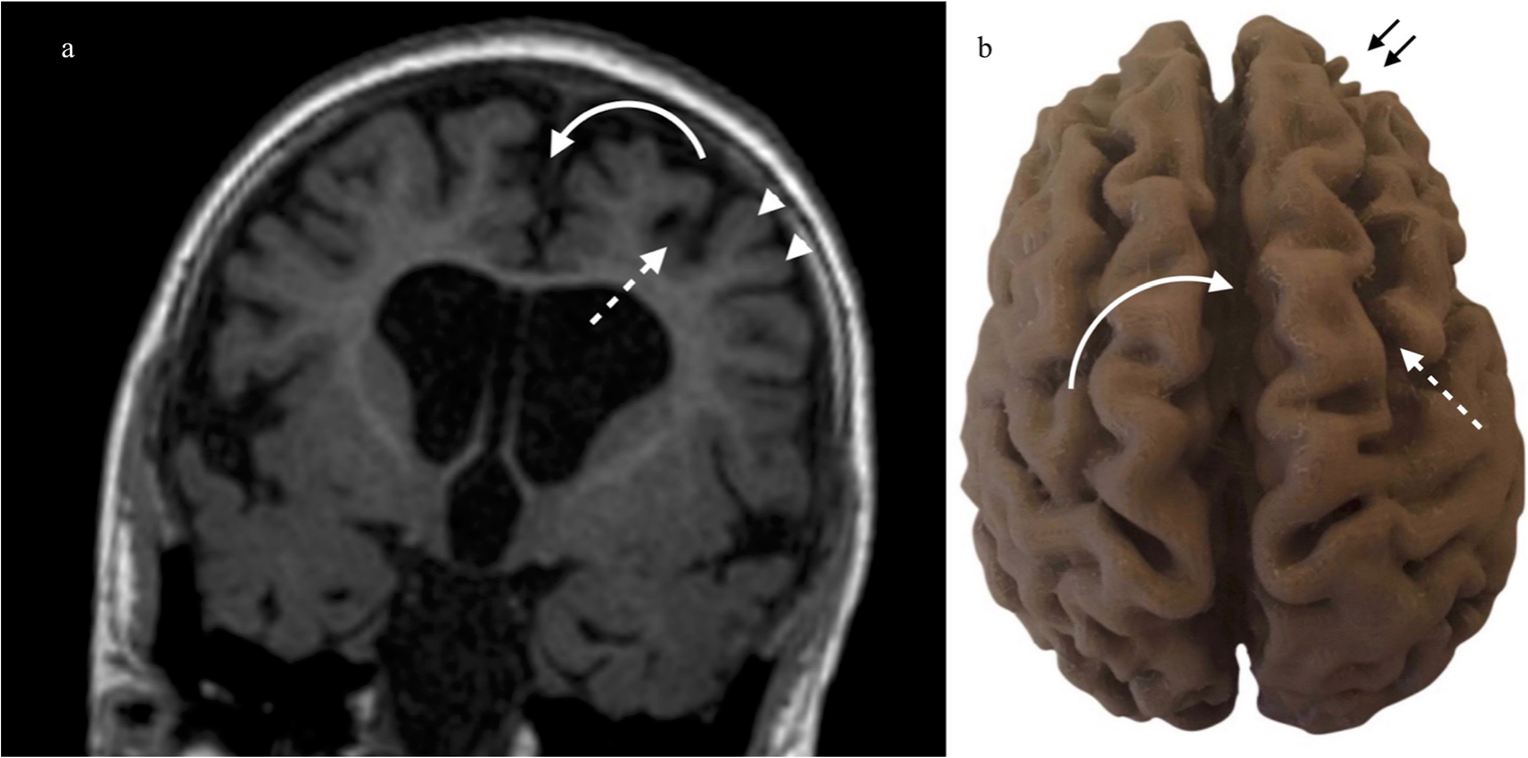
a, b Moderate/mild partial-prolonged hypoxic ischaemic injury to the brain with MR imaging at the age of 4 years and accompanying 3D print. a Coronal T1 weighted MRI at the level of the third ventricle demonstrates relatively mild changes of term partial-prolonged hypoxic ischaemic injury. Note widening of the interhemispheric fissure (curved arrow) and of the parasagittal sulci (dashed arrow) with ulegyria (arrowhead). b 3D printed model of the brain shown in Fig. 4a (mild changes of term partial-prolonged hypoxic ischaemic injury). There is moderate widening of the interhemispheric fissure (curved arrow). Note also prominent widening of the superior frontal sulcus corresponding to a parasagittal and anterior watershed continuum of volume loss (dashed arrow). Ulegyria is seen anteriorly (small black arrows)
